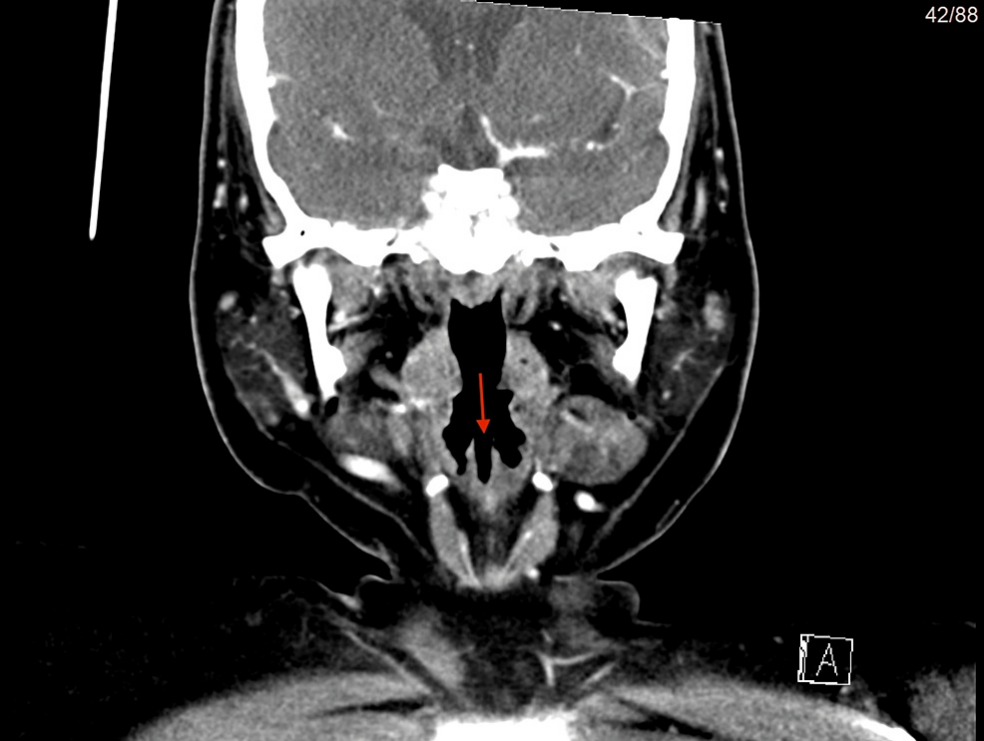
(CT scan) in coronal view The red arrow shows the bifid epiglottis with a cleft confined only to the upper one-third of the epiglottis, not reaching the base.
